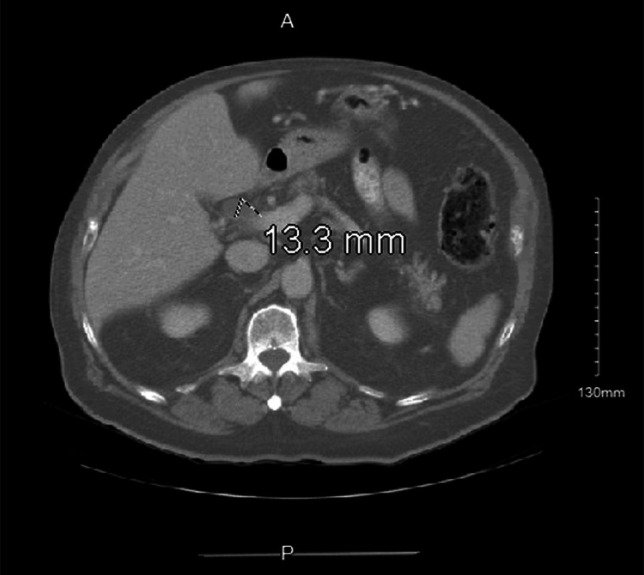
CT of abdomen and pelvis with IV and oral contrast depicting dilated CBD to 1.3 cm with an abrupt caliber change and concern for wall thickening. CBD, common bile duct; CT, computed tomography; IV, intravenous.
